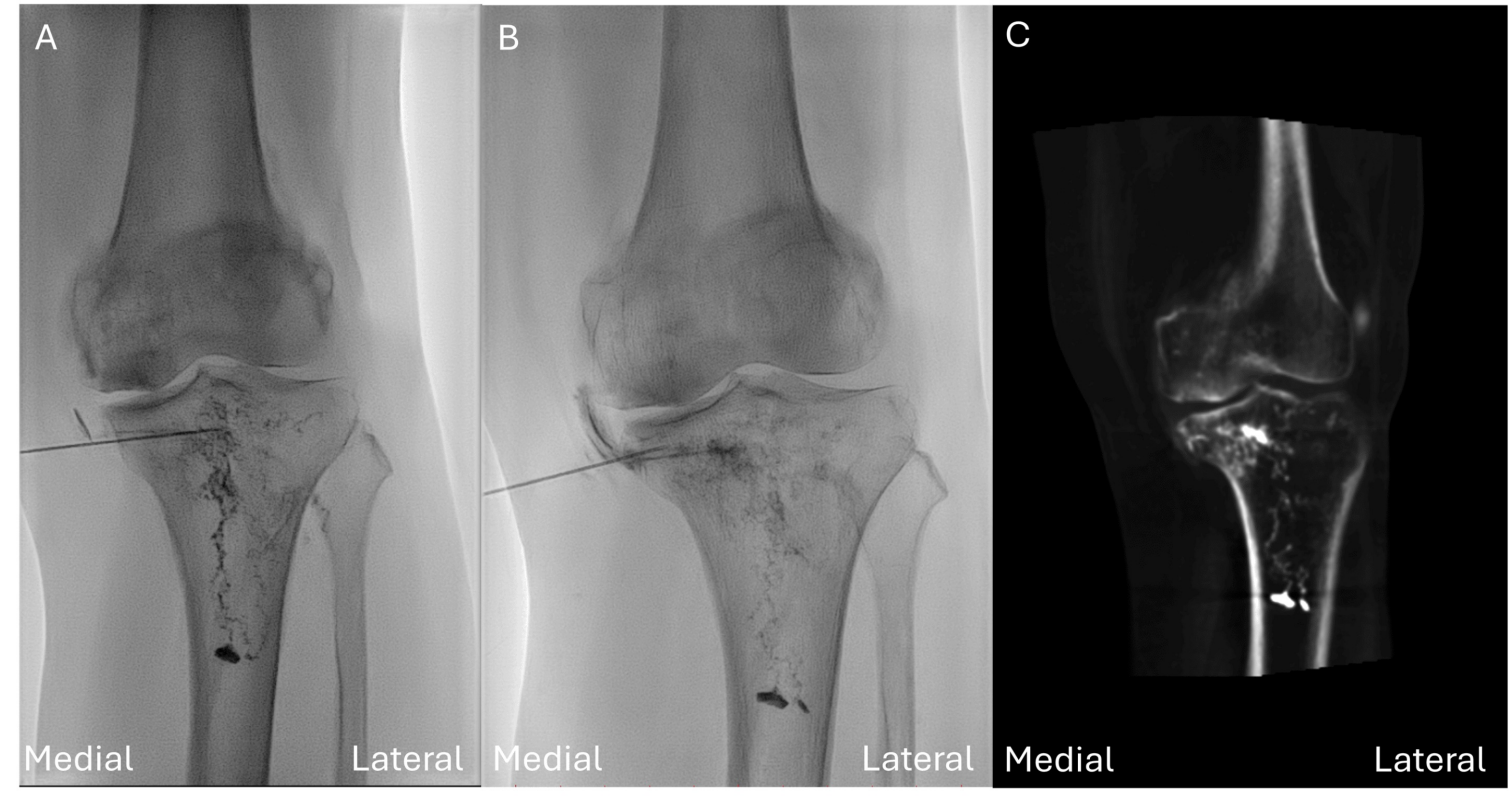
(A) Fluoroscopy showing a characteristic intramedullary intraosseous (IO) contrast medium pattern. (B) After needle withdrawal and repositioning towards the joint line, fluoroscopy demonstrated a subchondral contrast pattern. (C) Cone beam computed tomography (CBCT) confirming the subchondral spread pattern.
